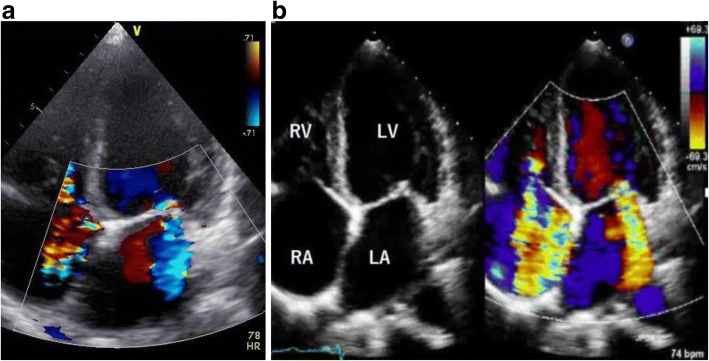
Color-Doppler Echocardiography of the mitral and tricuspid valves. There was a worsening of both mitral and tricuspid regurgitation in the last hospital admission (b) compared to at the end of previous hospitalization (a). RA, right atrium; RV, right ventricle; LA, left atrium; LV, left ventricle
